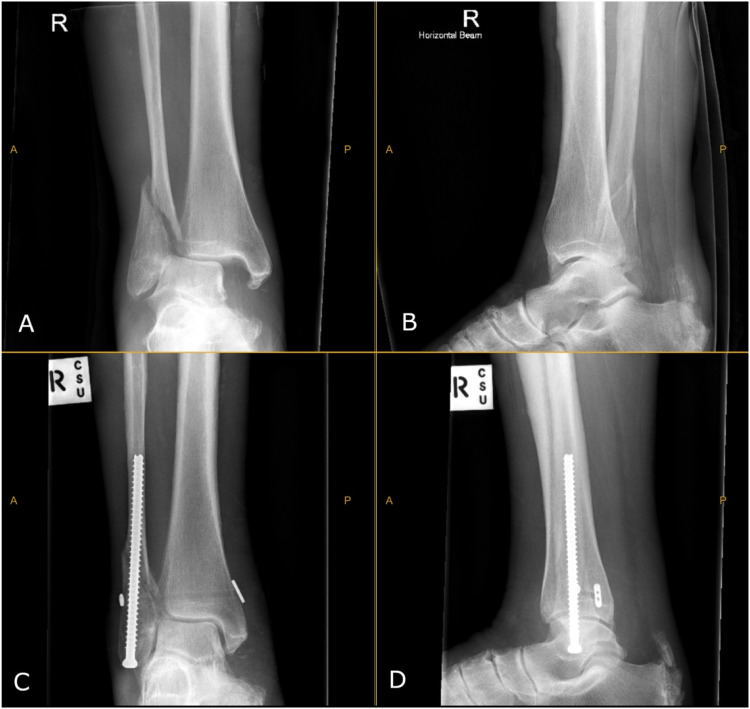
Radiographs of Case 9. Preoperative radiographs (A = mortise view; B = lateral view) demonstrate a subluxated ankle joint with a spiral distal fibula fracture. Postoperative radiographs at 6 weeks post operation (C = mortise view; D = lateral view) demonstrate a uniting fracture fixed with a 6.5mm cannulated screw in the fibula and a syndesmotic suture button
